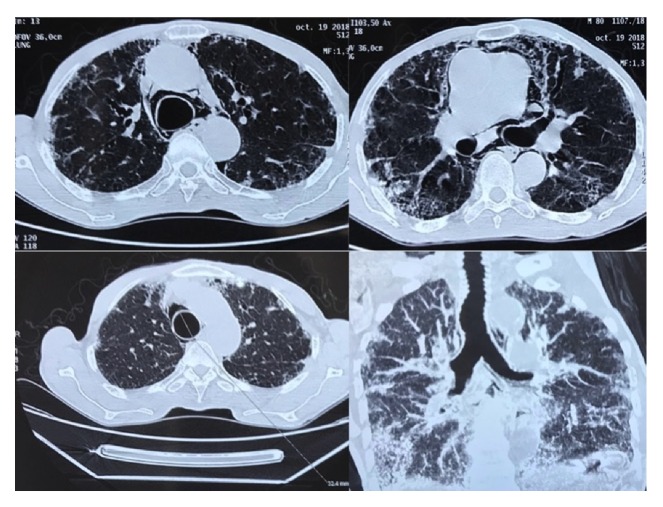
CT scan showing tracheal and both main bronchi dilation with associated pneumomediastinum.
